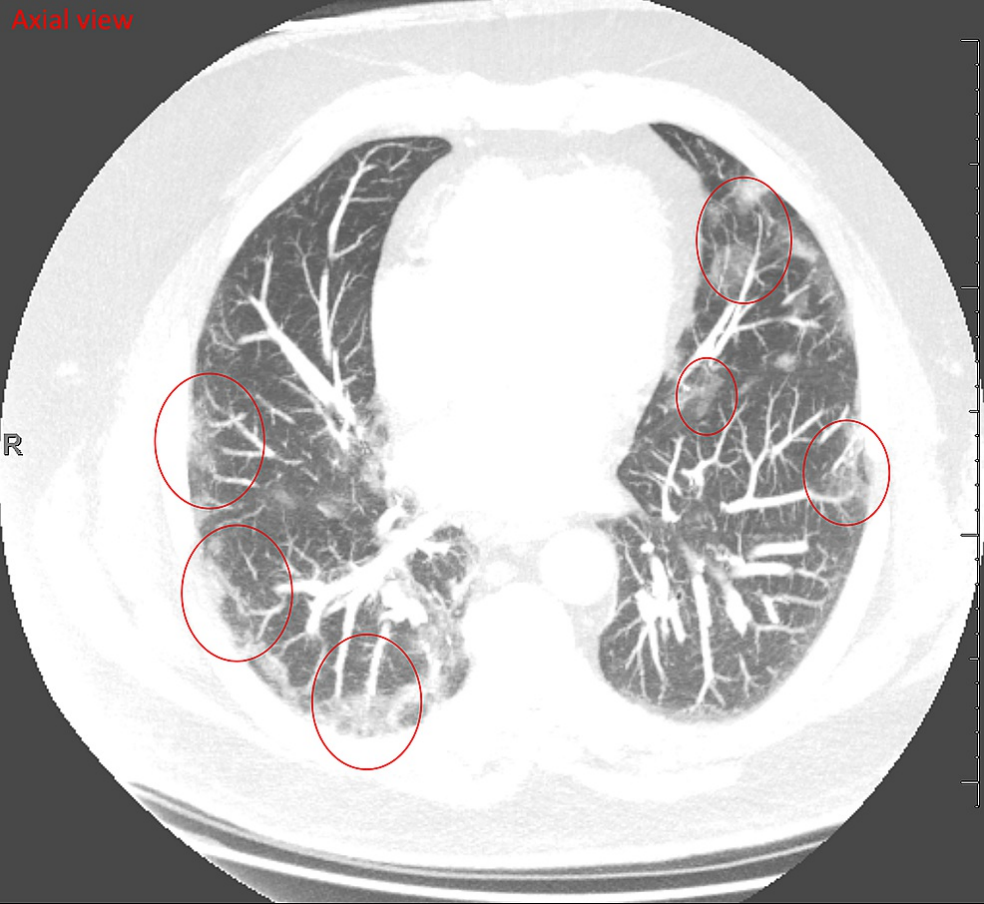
CT chest (axial view) Multilevel bilateral multifocal peripheral ground-glass opacities (red circles), suspicious for atypical pulmonary infection from bacterial and viral etiology, including COVID-19 CT: computed tomography; COVID-19: coronavirus disease 2019
